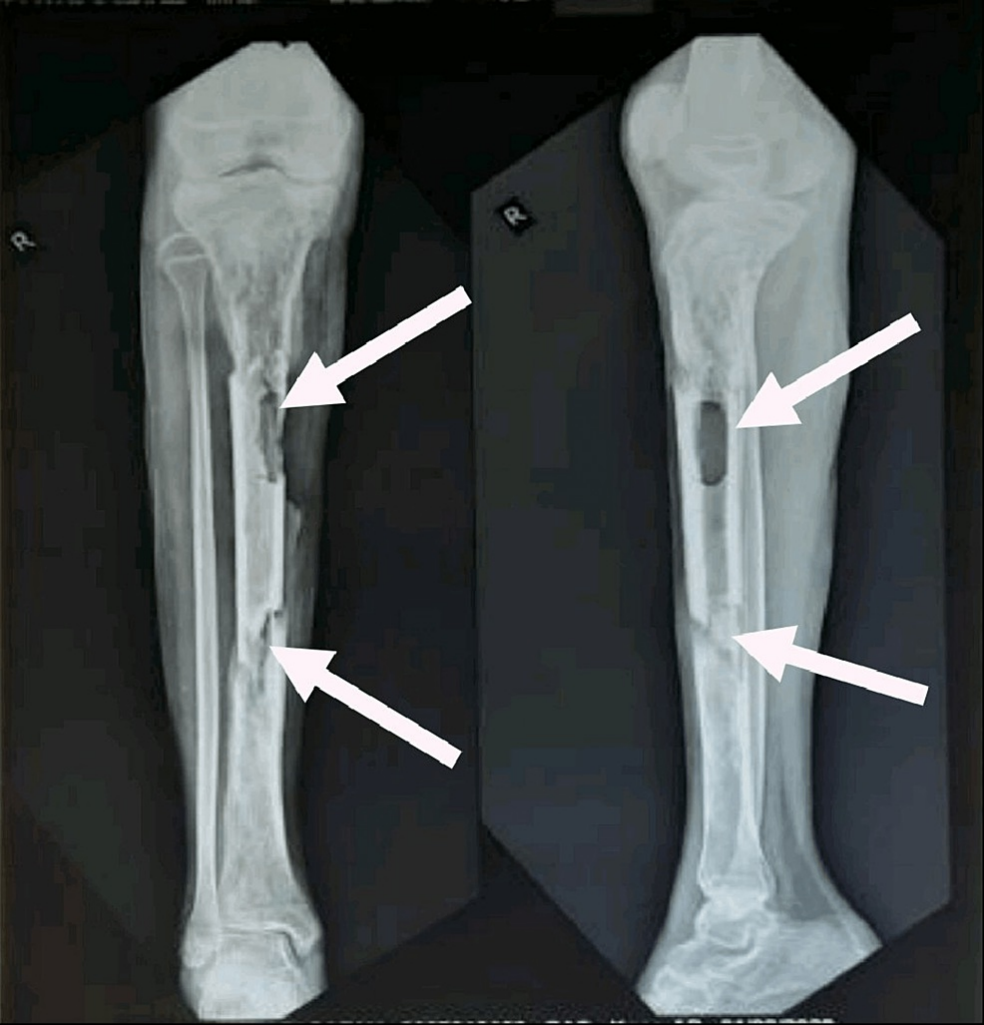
X-ray of the right lower leg (knee AP view) showing diffuse multiple osteolytic lesions with bone defect (sequestrum) mid tibia anteroposterior: AP
